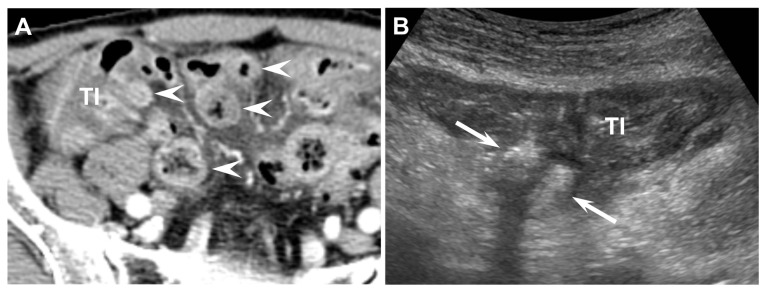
Seventy-eight-year-old male (patient #4) with acute ileal diverticulitis. (A) Enhanced axial CT scan shows multiple ileal diverticula (arrowheads) with or without fecalith, ileal wall thickening (TI), and mesenteric fat infiltration. (B) US scan with C5-1 convex transducer demonstrates two outpouching diverticular sacs (arrows) with central hyperechoic fecalith, ileal wall thickening (TI), and increased peridiverticular fat echogenicity.
